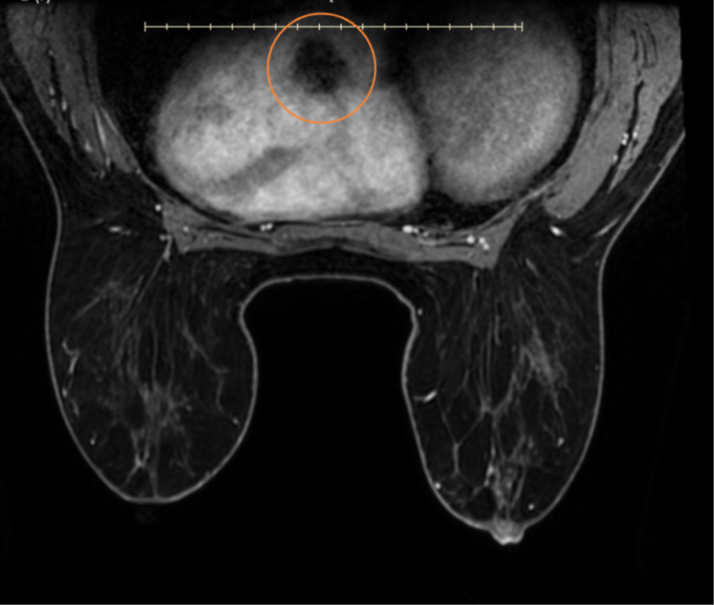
T1-Weighted Postcontrast Magnetic Resonance Imaging Breast magnetic resonance imaging: postcontrast T1-weighted gradient echo fat saturation sequence demonstrates a filling defect within the left atrium (orange circle), suggestive of a cardiac mass.
